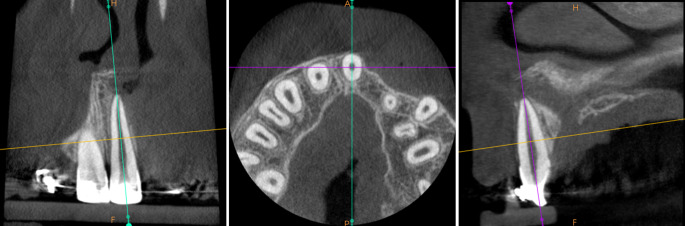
Standardized cone beam computed tomographic image Standardisierte DVT(digitale Volumentomographie)-Aufnahme
